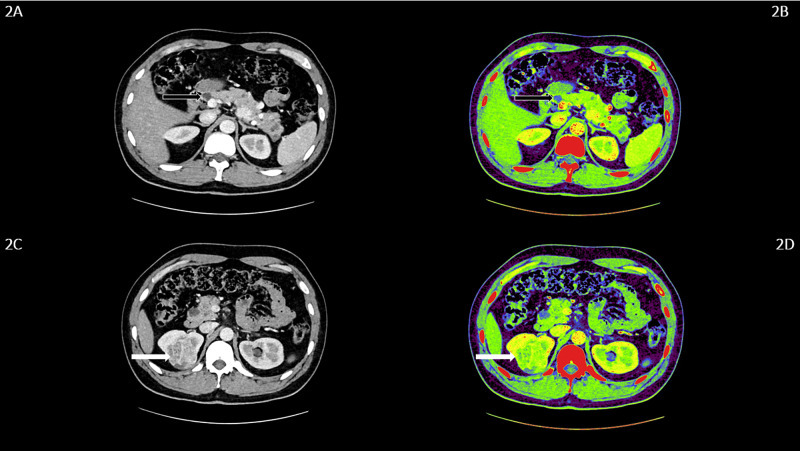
CECT whole abdomen of the asymptomatic patient demonstrating pancreatic and renal manifestations 2A and 2B: Axial CECT abdomen shows multiple small, simple cysts involving the entire pancreatic parenchyma (black arrows). 2C and 2D: Axial CECT abdomen shows a well-defined heterogeneously enhancing mass lesion, with few cystic areas within, arising from the upper pole of the right kidney, suggesting a neoplastic etiology (white arrows). CECT: contrast-enhanced computed tomography
